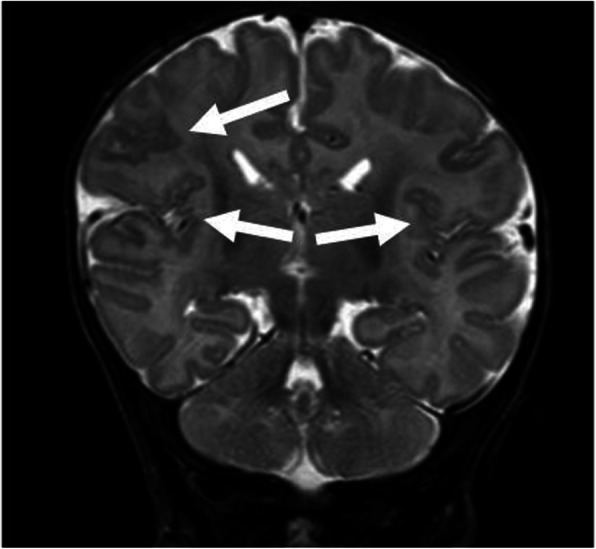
Polymicrogyria at brain MRI (T2-weigthed)
